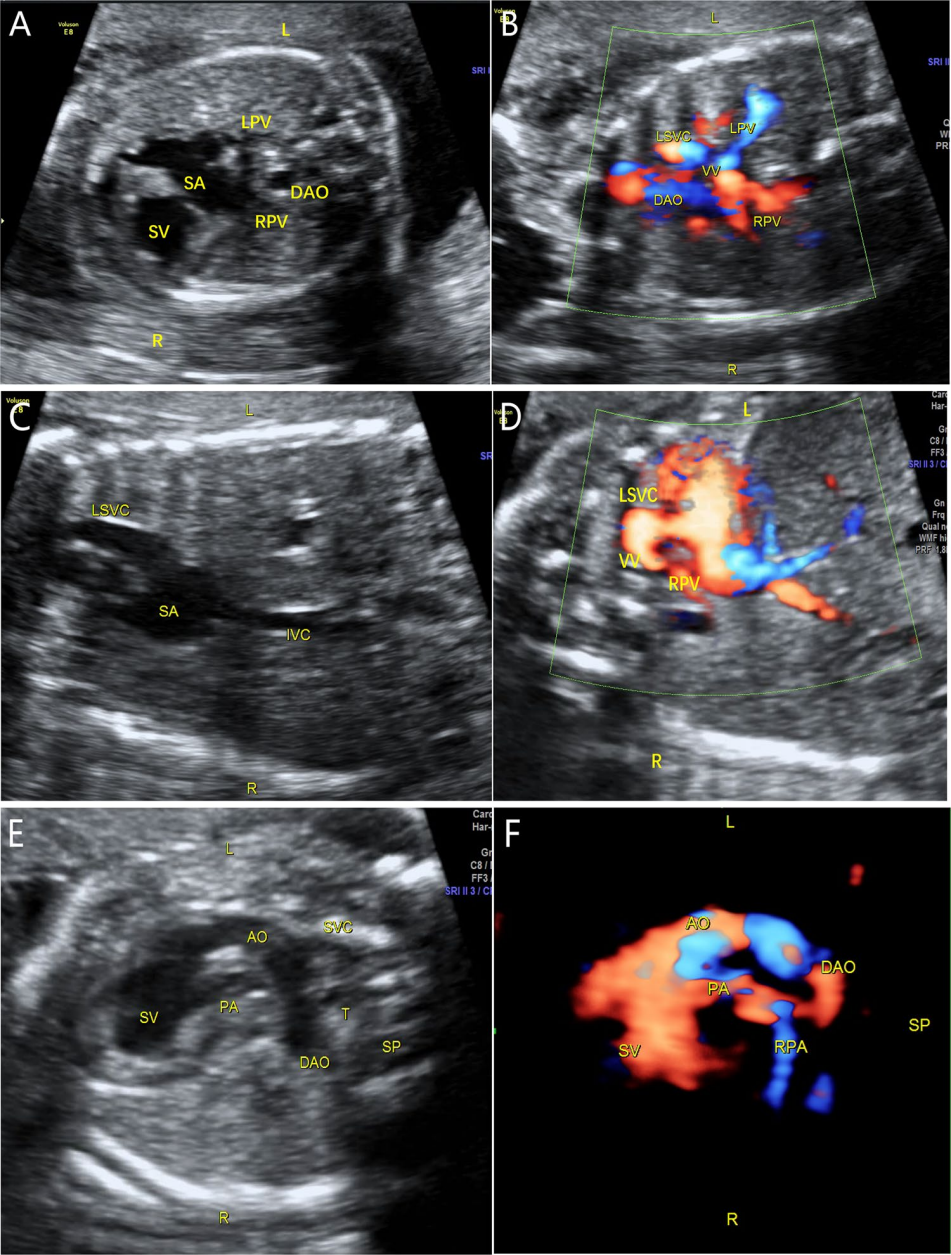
Prenatal ultrasound diagnosis of fetal supracardiac TAPVC with complex malformations. (A) The common PV trunk was visible in the cross section of the atrium, and posterior left atrium index was increased. (B) Vertical venous return to the left superior vena cava. (C, D) Superior and inferior vena cava return to a single atrium. (E, F) Two- and 3-dimensional flow imaging revealed both the aorta and pulmonary artery originating from a single ventricle with pulmonary stenosis. *DAO* descending aorta, *L* left, *LPA* left pulmonary artery, *R* right, *RPA* right pulmonary artery, *SA* single atrium, *SV* single ventricle.
